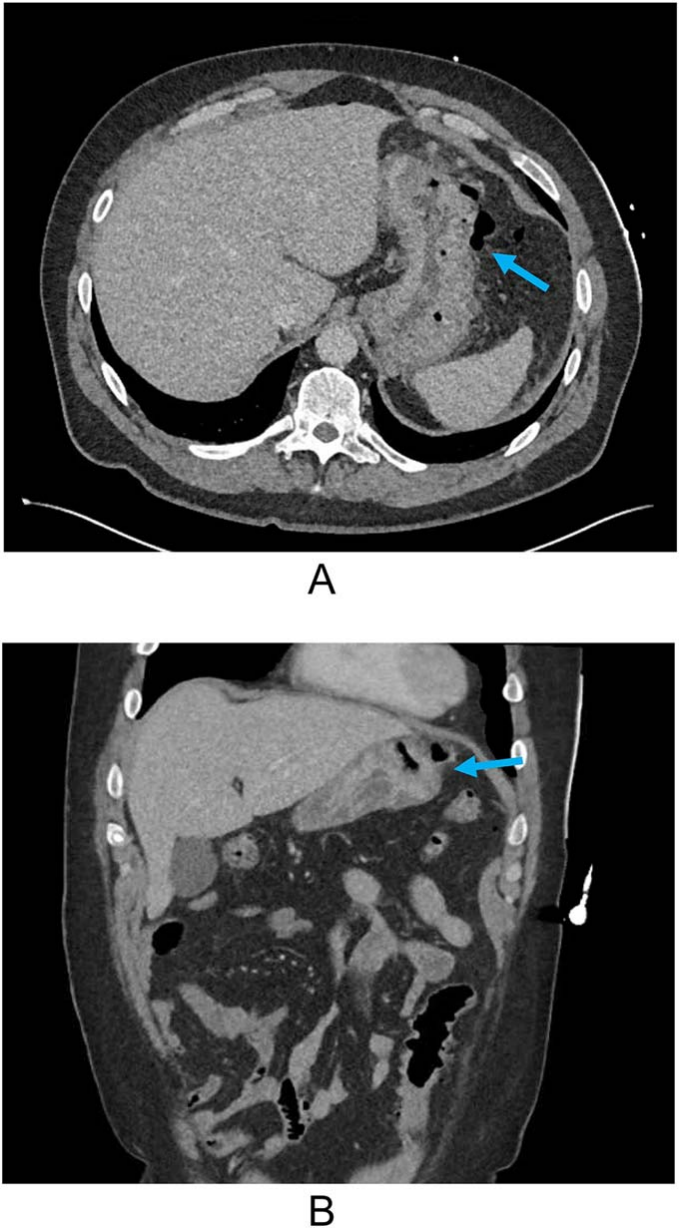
(A) Axial CT and (B) coronal reconstruction demonstrating perforated gastric ulcer at the greater region of the gastric body (arrows).
